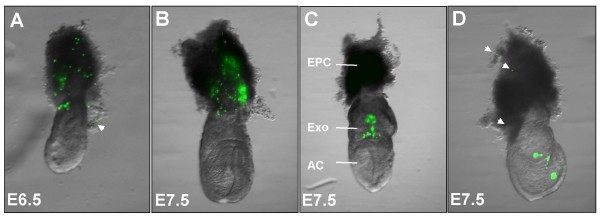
Target accuracy. Concepti dissected a few hours after ultrasound-guided microinjections of a 13.8 nL volume containing 3 μm diameter fluorescent beads. In these examples, there were few (e.g. arrows in A&D) or no beads considered off-target (e.g. B&C). The ectoplacental cone region was targeted in (A) at E6.5 and (B) at E7.5. At E7.5, the exocoelomic cavity (C) and amniotic cavity (D) were also targeted. AC, amniotic cavity; EPC, ectoplacental cone region; Exo, exocoelomic cavity.
